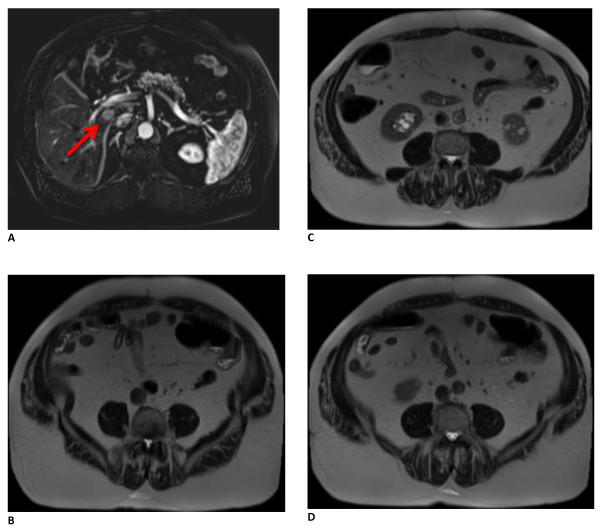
Cross-sectional magnetic resonance images of patient 30 months after biologic graft placement. A. Arrow indicates the recurrent carcinoid tumor in the liver. B-D. Additional caudad images demonstrating abdominal wall integrity.
